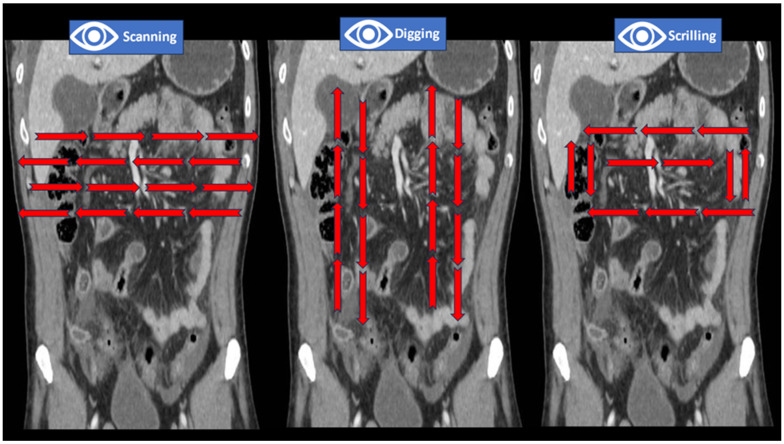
Techniques for multiple-target searching during CT examination. The red arrows show the direction of the visual search pattern.
